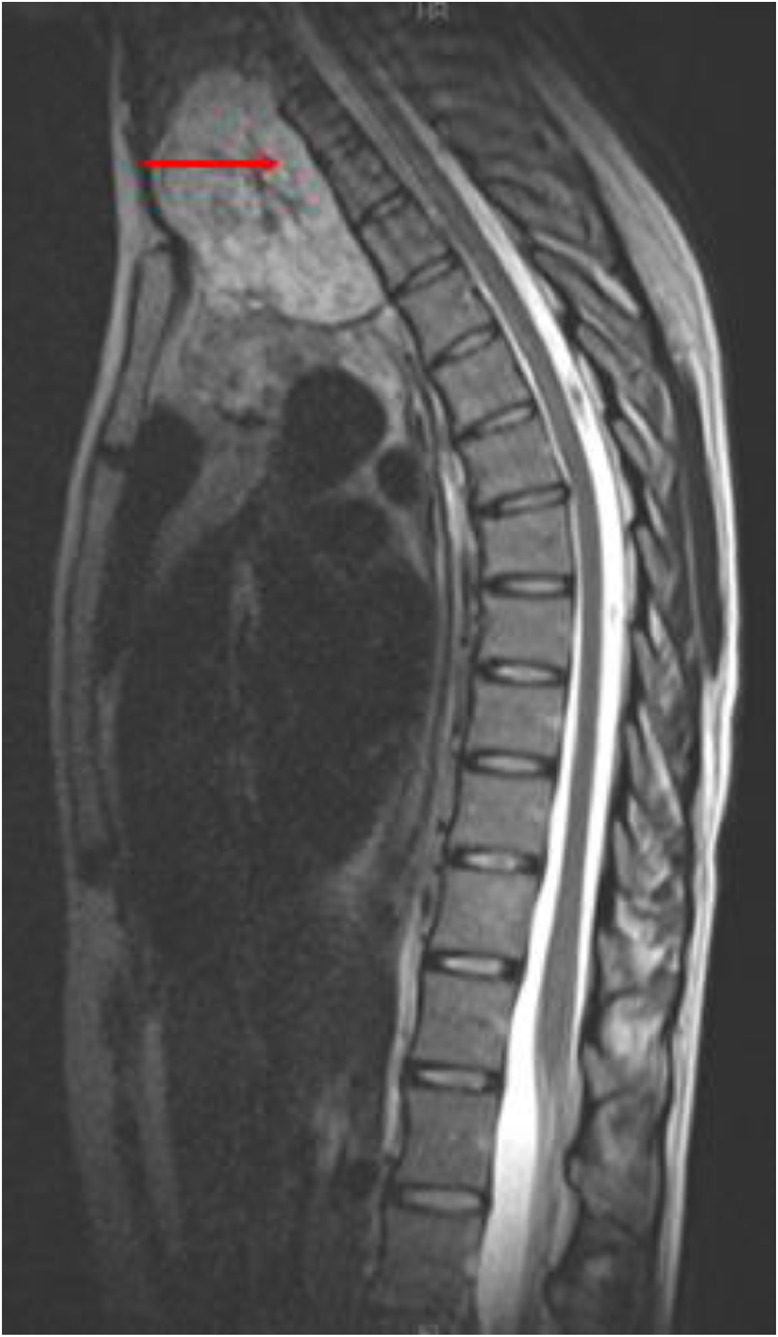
MRI Spine demonstrates heterogenous T2 lesion with enhancement in the upper mediastinum (red arrow) that abuts the ventral surface of the cervical spine without signal abnormalities of the vertebral body. (Color version of figure is available online.)
